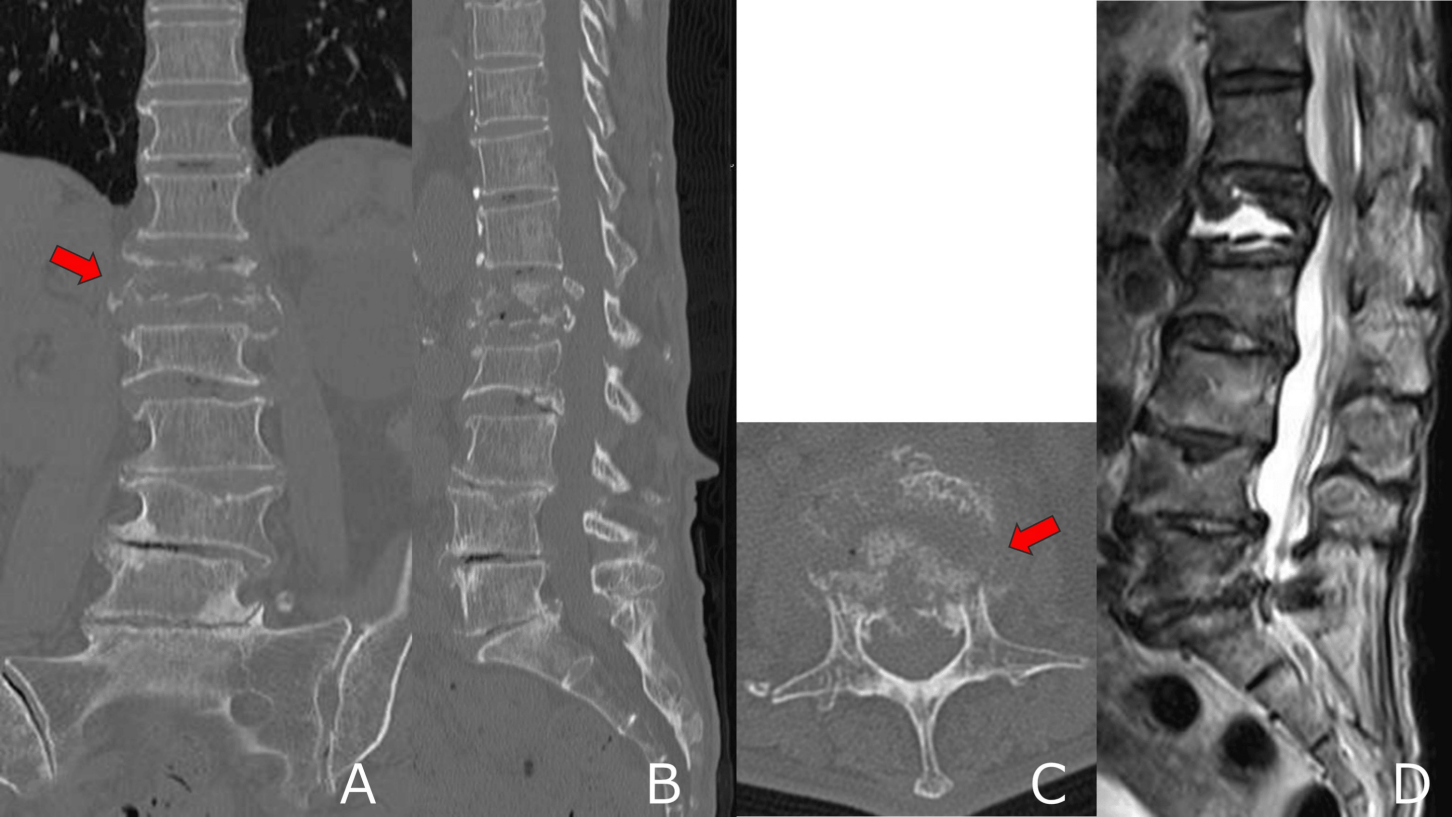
Preoperative computed tomography (CT) and magnetic resonance imaging (MRI). (A-C) CT images showing a vacuum cleft at L1. Bone cortical continuity of the vertebral body is not clear (arrow). (D) Sagittal T2-weighted MRI showing fluid retention in L1.
